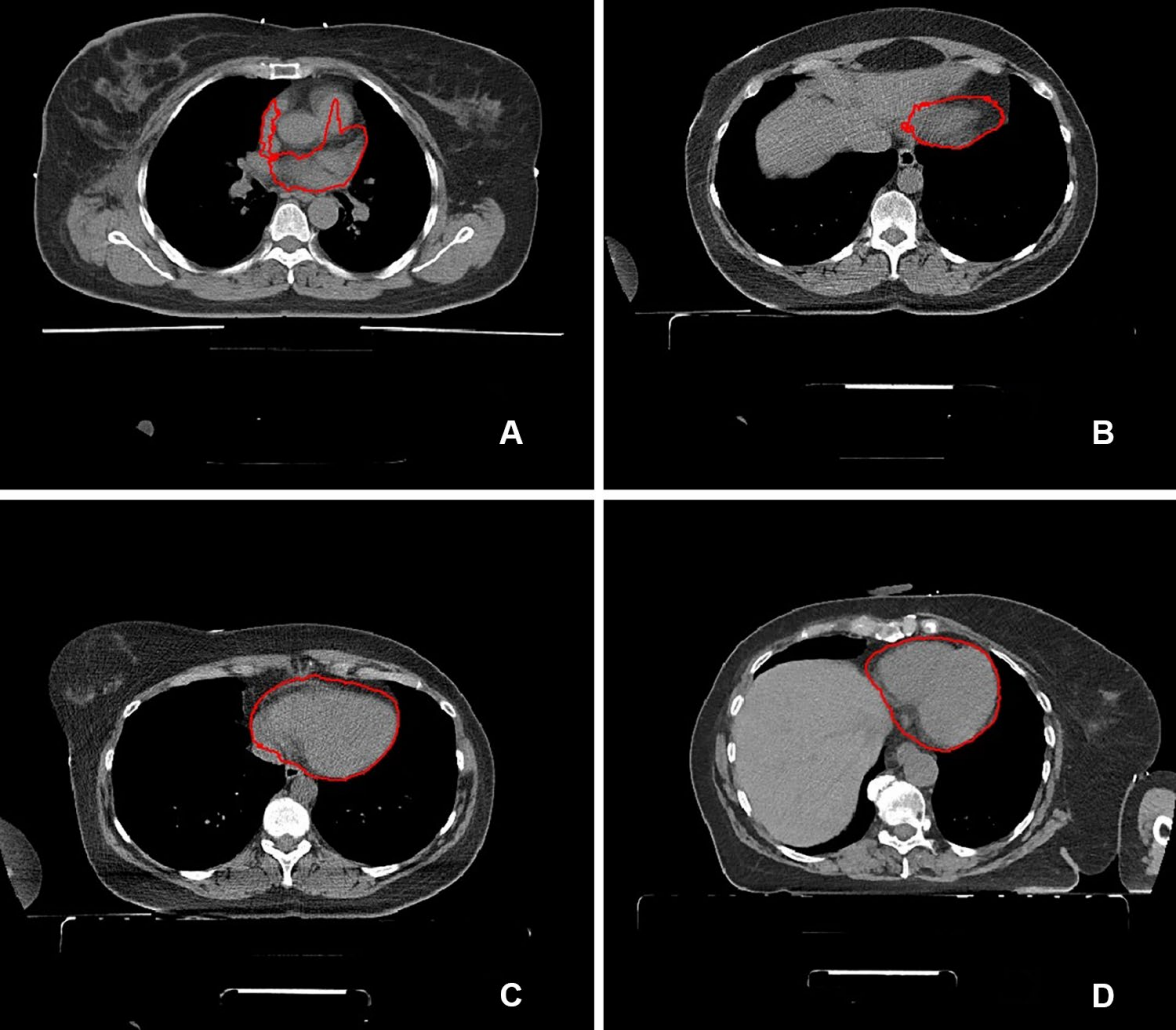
4 CT slices with predicted contouring of the heart as shown to the radiologists for the qualitative evaluation. The letters correspond with the consensus answers for this particular slide. A was rejected with clear mistakes, B was rejected with minor but clinically relevant mistakes, C was accepted with small but irrelevant mistakes and D was accepted with no mistakes
